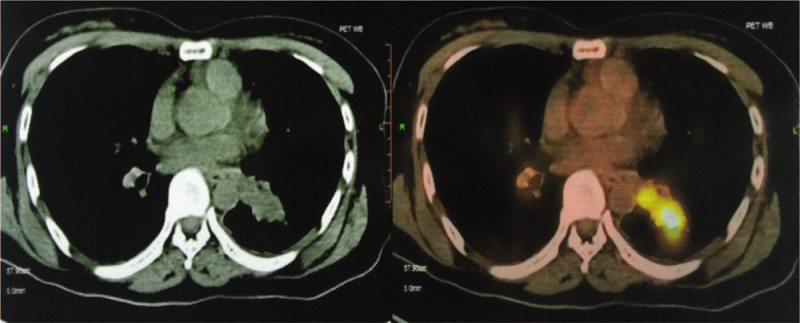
Whole body PET-CT showed that fusiform soft tissue shadows with a size of about 43 mm × 22 mm × 32 mm could be seen near the hilum of the lower lobe of the left lung. Glucose metabolism was increased and the edges were lobulated. Malignant lesions were mainly considered.
